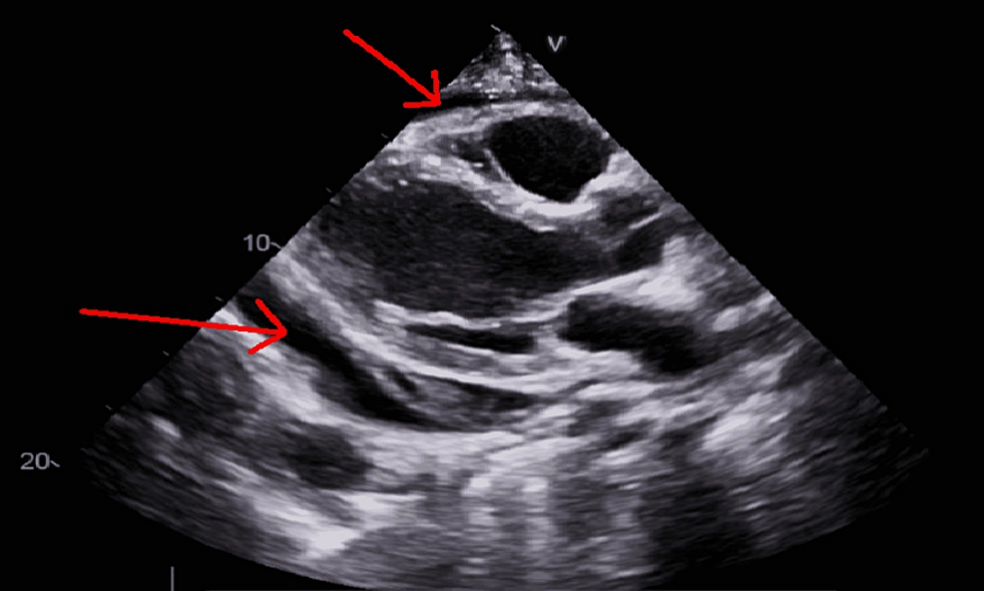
Transthoracic echocardiogram on September 2021. Red arrows point to large pericardial effusion.
